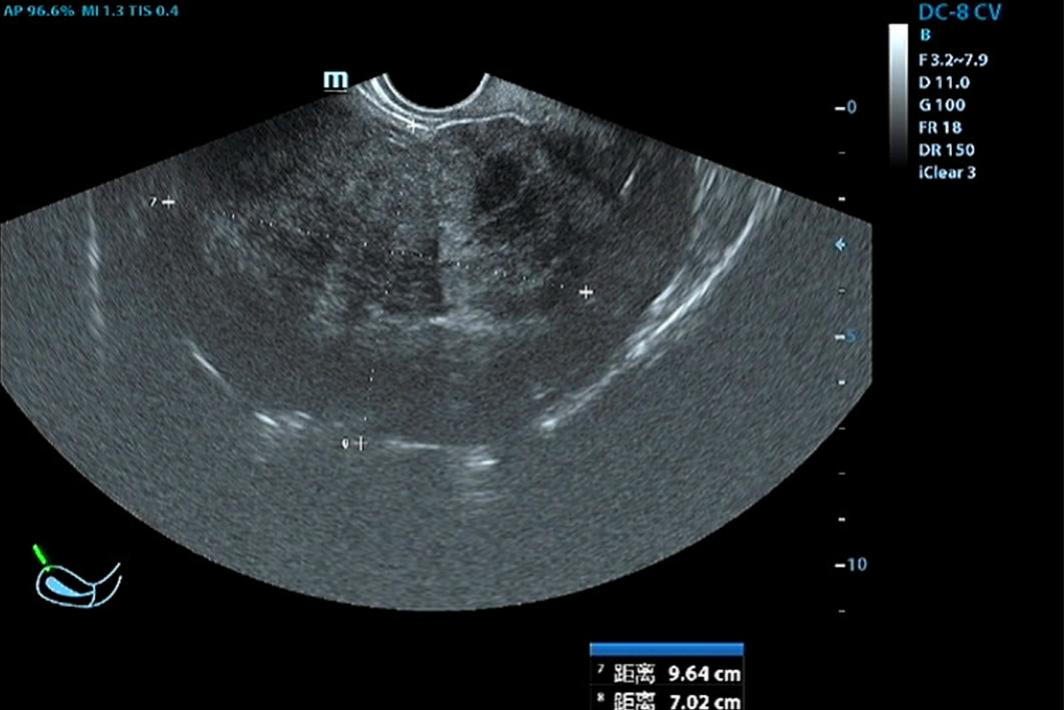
Vaginal Doppler Ultrasonography: Reveals a large pelvic mass measuring 9.6 × 7.0 × 10.8 cm, indicative of a significant space-occupying lesion.
